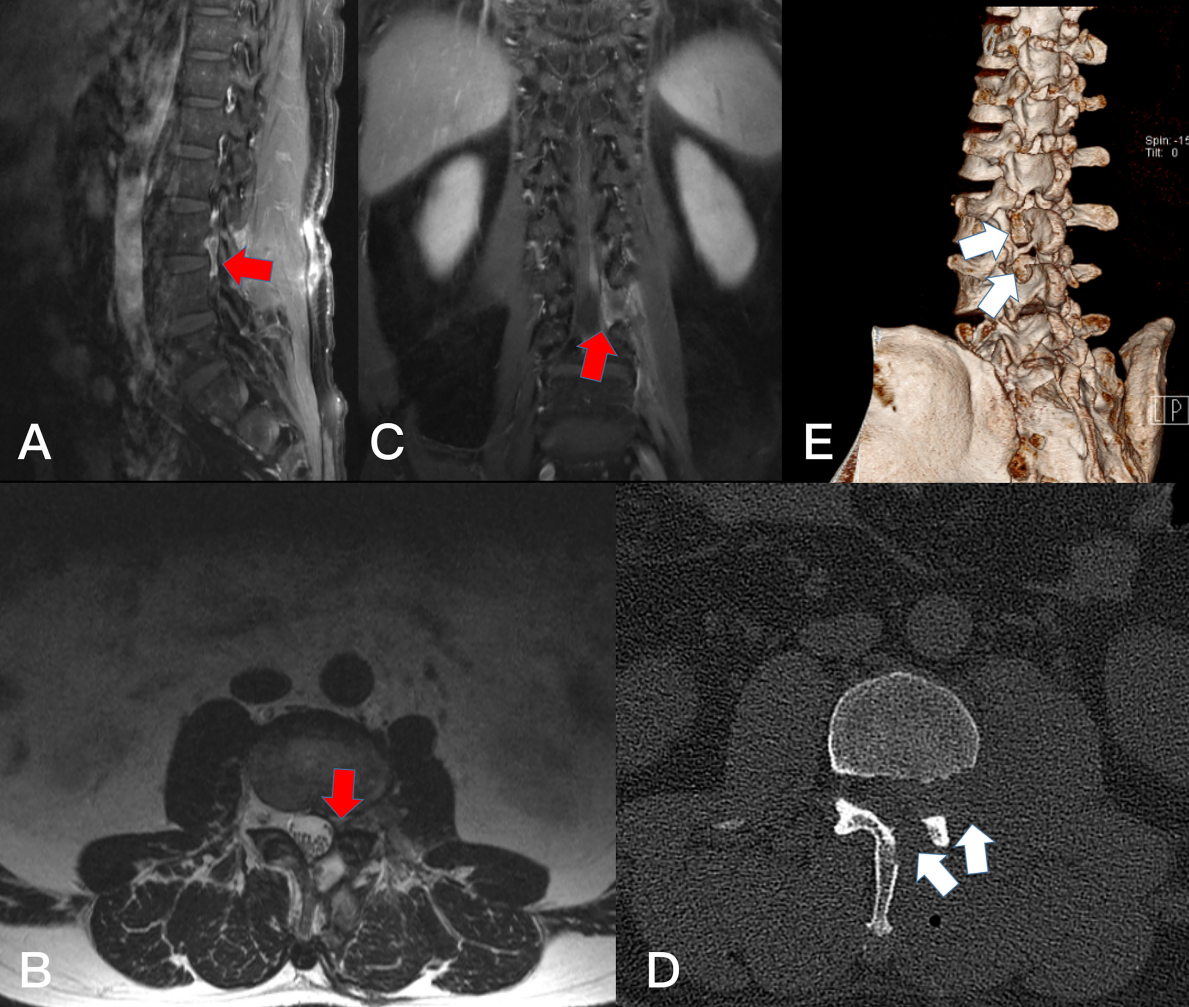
Postoperative T2-weighted MRI on sagittal (A), axial(B), and T1-weighted enhanced on coronal (C) plane, Resection of the dumbbell tumor could not be identifiedafter 6 months (red arrows). The axial plane and 3D-CT scan showed the bone window of L3 and L4 lamina (D, E) (white arrows).
